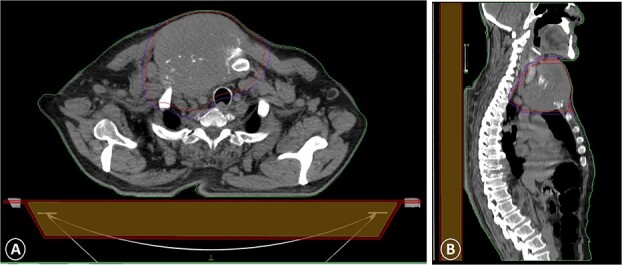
A CT scan with intravenous contrast that showed a sternal tumor of 11 by 12 cm: (A) transversal plane and (B) sagittal plane.
